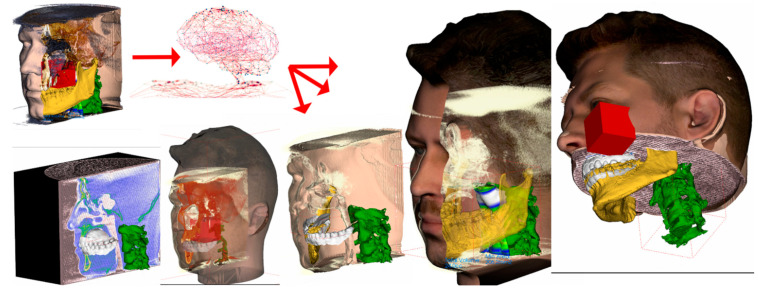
The example of 3D data augmentation in a sense of mapping another 3D scans on the segmented structures. Facial 3D scan with texture mapped on the segmented face surface from CBCT and intraoral scan of teeth and gums mapped on tooth surfaces from the CBCT. Finally merged into complex set of 3D models. Training of 3D CNN with such a complex 3D virtualized model has never been performed before and is worth a consideration.
